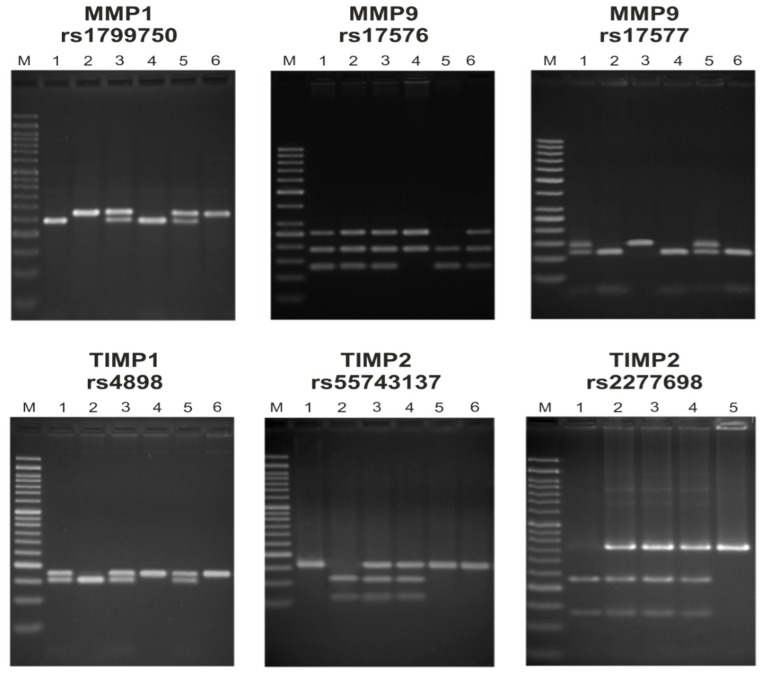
Examples RFLP reaction results: rs17577 MMP1 (lines 1, 4—1G/1G, 3, 5—1G/2G, 2, 6—2G/2G), rs17576 MMP9 (4—AA, 1, 2, 3, 6—AG, 5—GG), rs17577 MMP9 (2, 4, 6—GG, 2, 5—GA, 4—AA), rs4898 TIMP1 (4, 6—TT, 1, 3, 5—TC, 2—CC), rs2277698 TIMP2 (5—CC, 2, 3, 4—CT, 1—TT), rs55743137 TIMP2 (1, 5, 6—TT, 3, 4—TG, 2—GG), Lane M—50 bp marker used as molecular-weight reference in each gel. Lane numbers correspond to representative genotypes. The above RFLP analysis was performed for qualitative genotyping.
